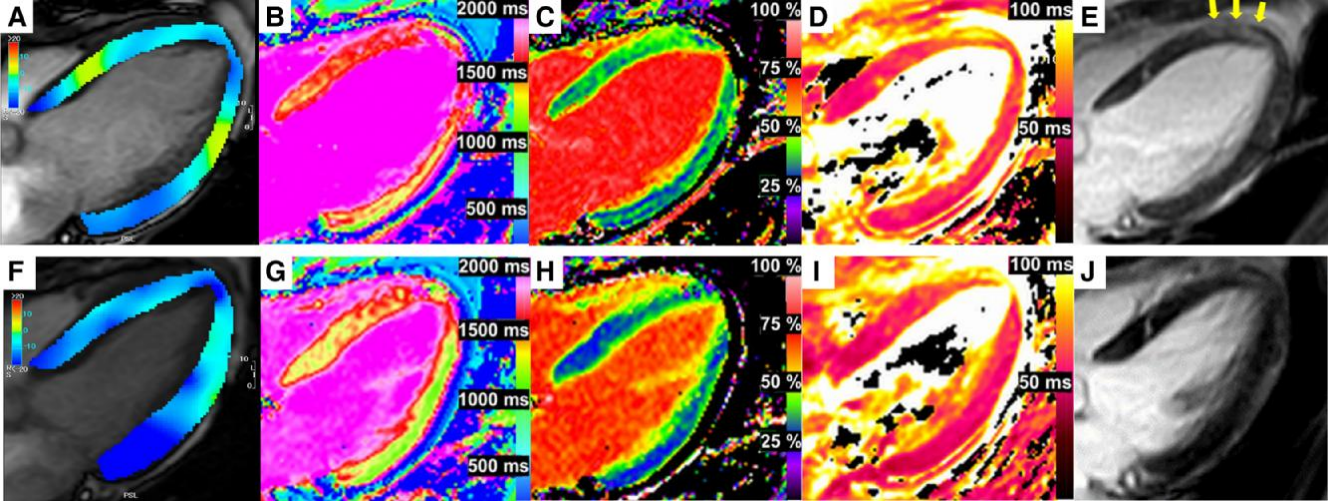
Cardiac magnetic resonance abnormalities and recovery. Cardiac magnetic resonance imaging on Day 4 (*A–E*) and Day 59 (*F–J*). A coloured strain analysis (Circle CVI42®) with left ventricular mid-ventricular hypokinesis (*A*) and recovered wall motion (*F*). Increased native T_1_ (*B*), extracellular volume fraction (*C*), and T_2_ (*D*) on Day 4 and normalized native T_1_ (*G*), extracellular volume fraction (*H*), and T_2_ (*I*) on Day 59. Focal late gadolinium enhancement (arrow) at baseline (*E*) and its disappearance in the follow-up (*J*).
